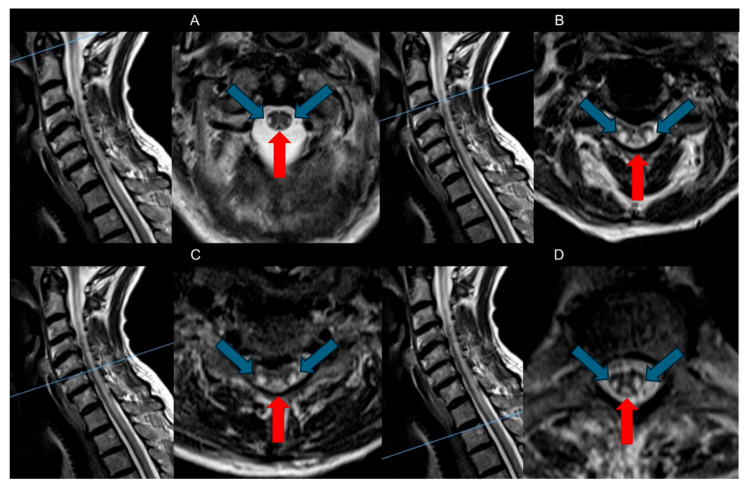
A 74-year-old woman presented with transient facial weakness and speech difficulties, alongside a history of hypertension, diabetes, and cognitive decline. Initial imaging ruled out acute cerebrovascular events, but persistent symptoms prompted further investigation with MRI of the spinal cord showed in the figure. Each image displays a T2-weighted sagittal image on the left, indicating the anatomical level of the lesion, and a corresponding T2-weighted axial image on the right, demonstrating the specific involvement of the spinal cord columns with the characteristic pattern of SCD. MRI revealed bilateral hyperintensities in the cervical and upper thoracic regions, in the dorsal column (red arrows) and the lateral columns (blue arrows), consistent with subacute combined degeneration (SCD) due to severe vitamin B12 deficiency, confirmed by lab tests. The levels shown are: (A) Axial view at the C2 vertebral level; (B) Axial view at the C3-C4 vertebral level; (C) Axial view at the C5-C6 vertebral level and (D) Axial view at the T1-T2 vertebral level. Treatment with high-dose vitamin B12 was initiated to prevent further spinal cord damage. (SCD: subacute combined degeneration).
